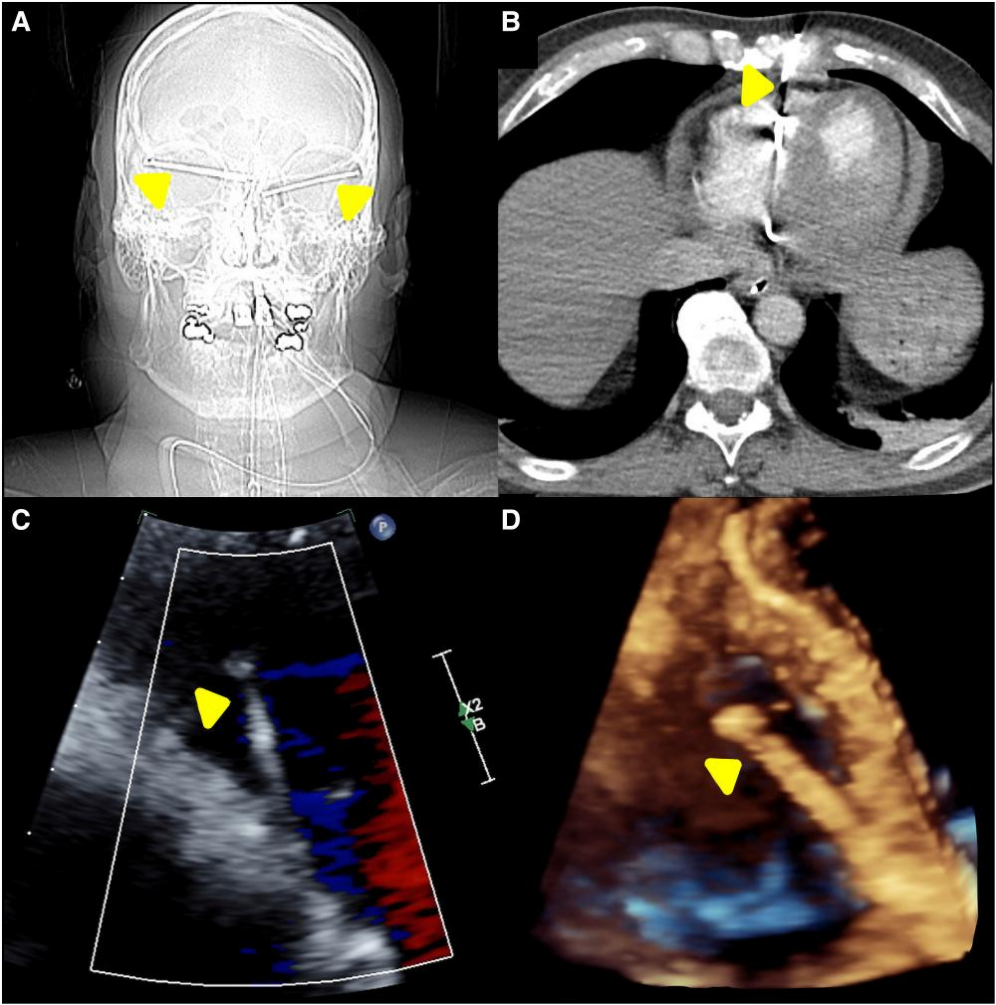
(A)* Radiographic image of multiple nails traversing the bilateral orbits and penetrating intracranially. Two nails are seen entering through the right temple, with one terminating in the medial right orbit. Another nail enters through the left temple and terminates in the medial left orbit (arrows). *(B)* Chest CT demonstrating a single nail penetrating the right ventricle. The nail is seen traversing the chest wall, breaching the pericardium, and embedding within the right ventricular cavity (arrow). *(C)* Two-dimensional transthoracic echocardiogram showing a nail piercing the right ventricle. The nail penetrates the right ventricular free wall, with its head protruding into the ventricular cavity (arrow). *(D)* Three-dimensional transthoracic echocardiogram depicting a nail lodged within the interventricular septum. The nail remains embedded within the septum, with its head visible within the ventricular cavity (arrow).
